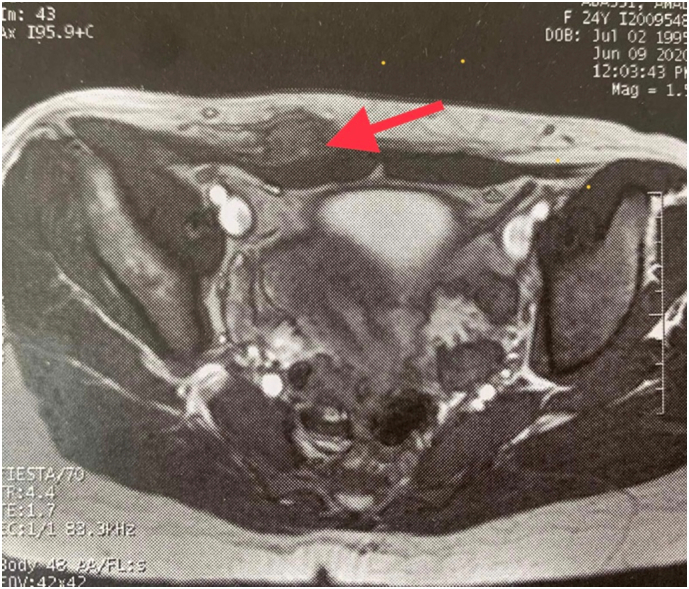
Abdominal wall endometriosis MRI signs: a hyperintense heterogeneous mass on both T1 and T2-weighted sequencies (red arrow). (For interpretation of the references to colour in this figure legend, the reader is referred to the web version of this article.)
